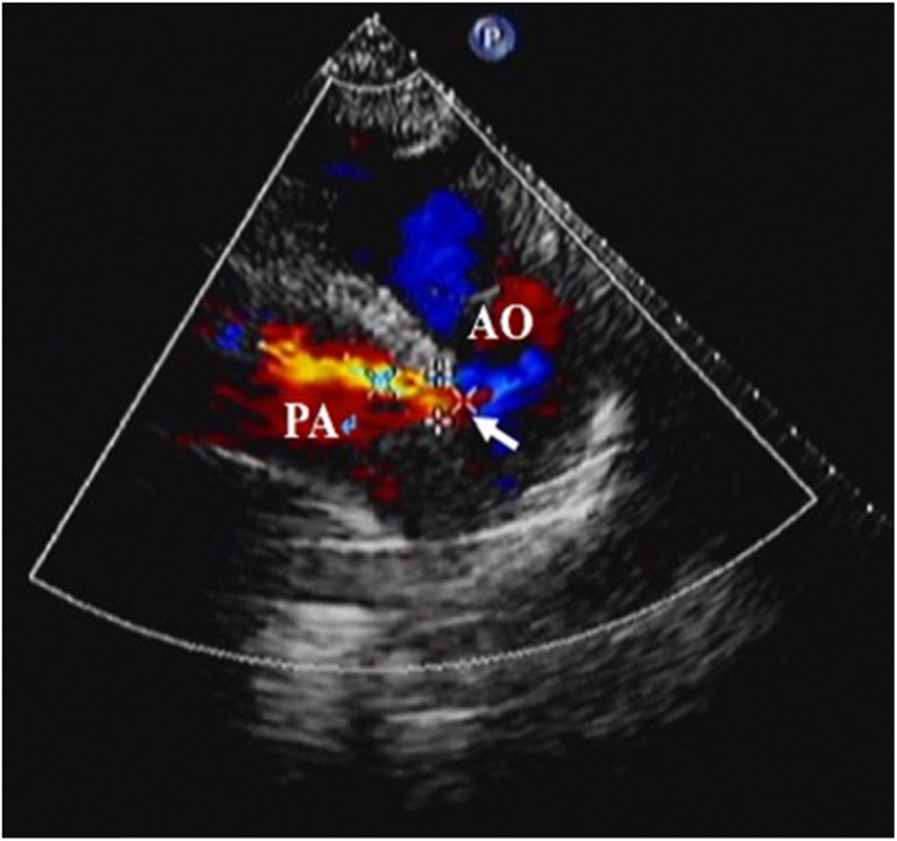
Transthoracic echocardiography results before occlusion. The white arrow indicates the patent ductus arteriosus. PA, pulmonary artery; AO, aorta
